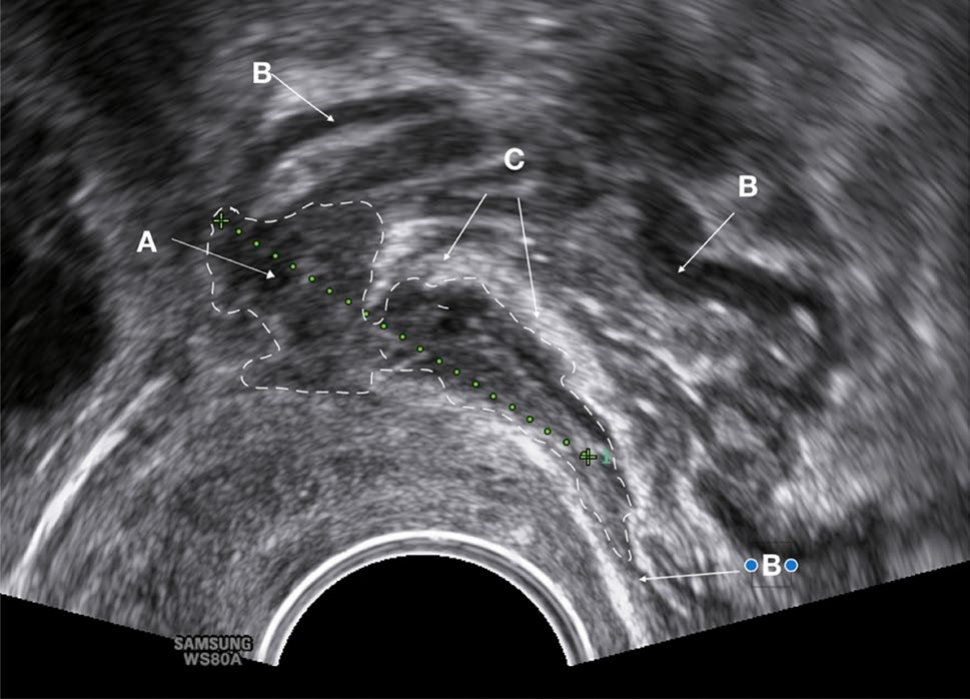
Sonographic image of an irregular deep endometriosis nodule in the rectal anterior wall (length 1.8 cm = #Enzian(u)C2). The hypodense area (A) represents the marked widening of the muscle layer due to a deep endometriosis nodule, accompanied by fibrosis or myohyperplasia of the layer. The normal pattern of the muscle layer is visible in the caudal direction and the parts of the posterior wall (B). The lesion lies directly underneath the mucosa (full thickness defect) (C)
